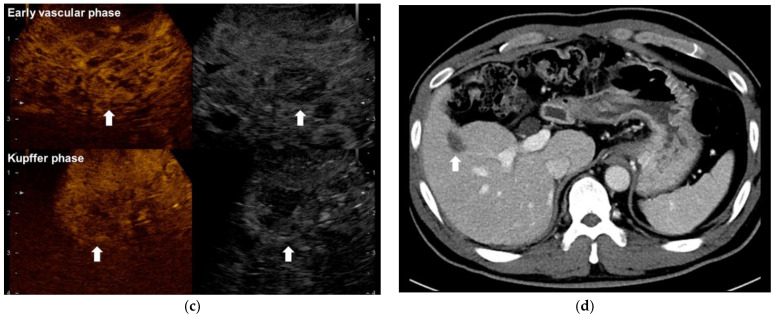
Images of a 39-year-old male patient with intermediate-stage HCC who underwent left lateral sectionectomy plus IORFA. (a,b) Contrast-enhanced CT images in the arterial phase, showing (a) a 3.6 cm HCC in liver segment 2 (black arrow), which was resected, and (b) a 1.2 cm HCC in liver segment 5 (white arrow), which could not be resected due to insufficient future liver remnant. (c) Sonazoid-enhanced US images during IORFA showing arterial enhancement with a Kupffer defect in liver segment 5 (white arrows). (d) CT image 8 years later showing no evidence of a viable HCC in the remnant liver with an RFA defect (white arrow).
